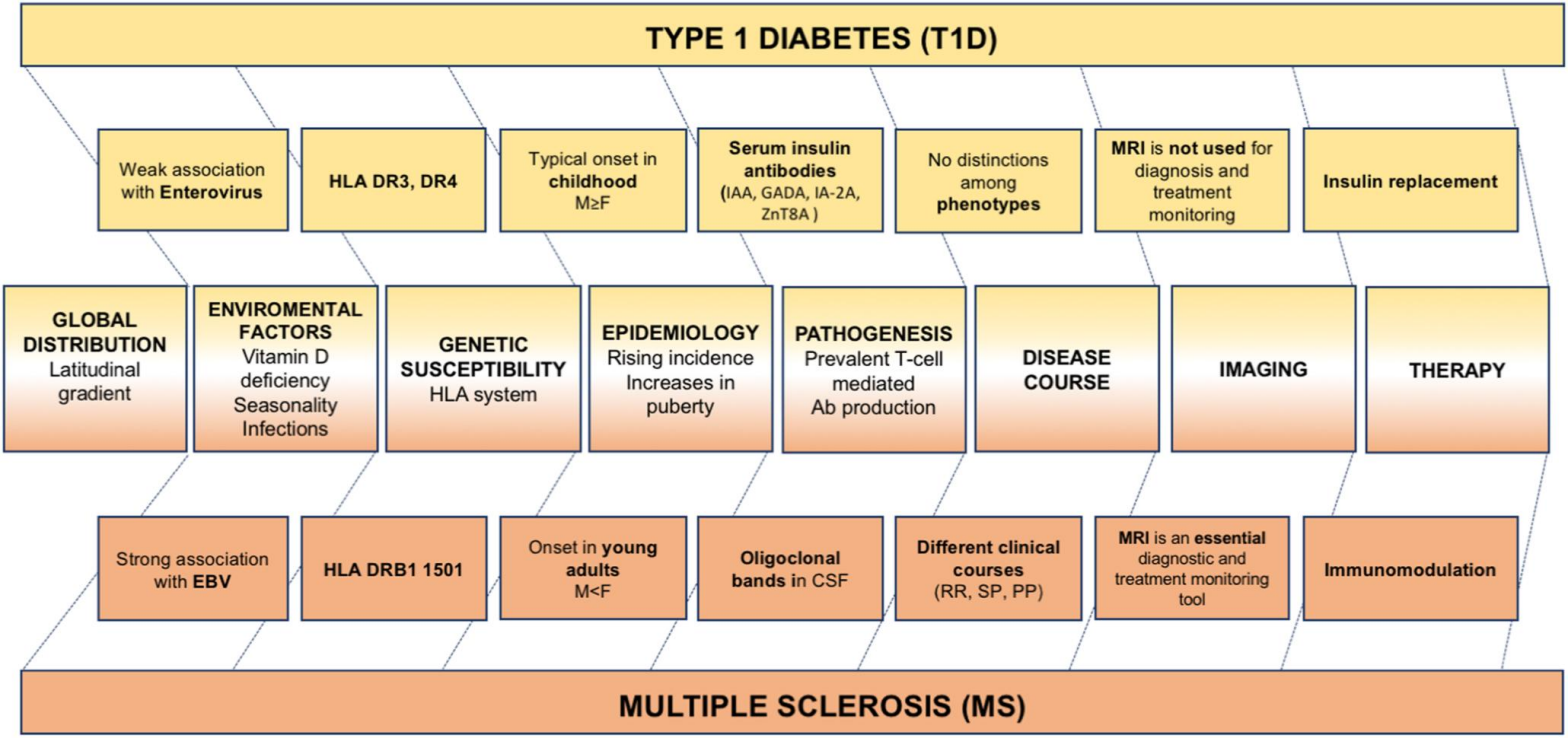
Similarities and differences between type 1 diabetes and multiple sclerosis. The middle row shows commonalities between the two conditions; differences or characteristics specific to one condition only are reported in the rows close to the each of the conditions. CSF, cerebrospinal fluid; CT, computed tomography; EBV, Epstein–Barr virus; GADA, glutamic acid decarboxylase antibody; HLA, human leucocyte antigen; IA‐2A, islet antigen 2 autoantibody; IAA, insulin autoantibodies; MRI, magnetic resonance imaging; PP, primary progressive; RR, relapsing remitting; SP, secondary progressive; ZnT8A, zinc transporter 8 autoantibody
